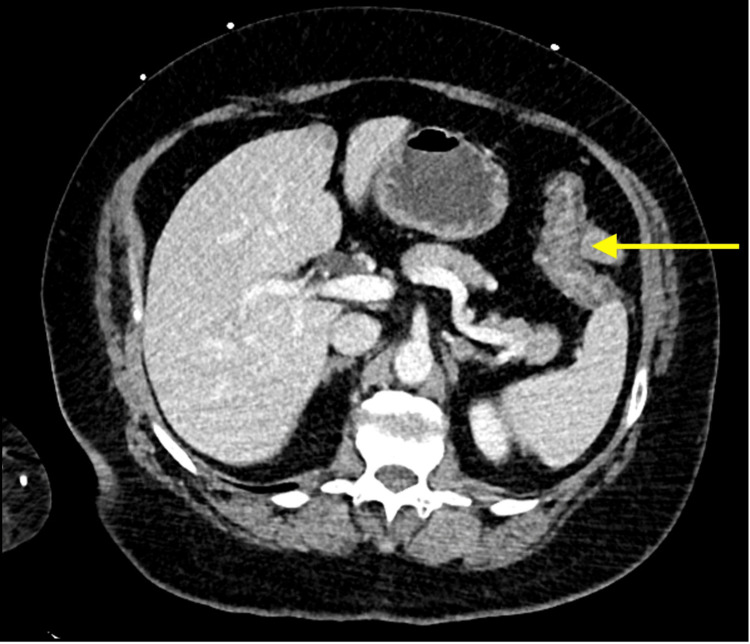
CT scan showed thickened colonic wall (yellow arrow)
